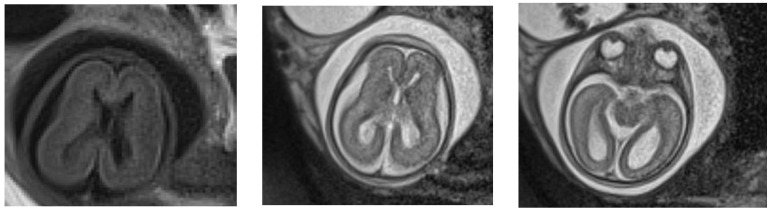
MRI at 24 weeks of gestation: no Sylvian fissure are seen; ventricular dilatation; the parieto-occipital and Sylvian fissures appear flat and the subarachnoid space is increased.
